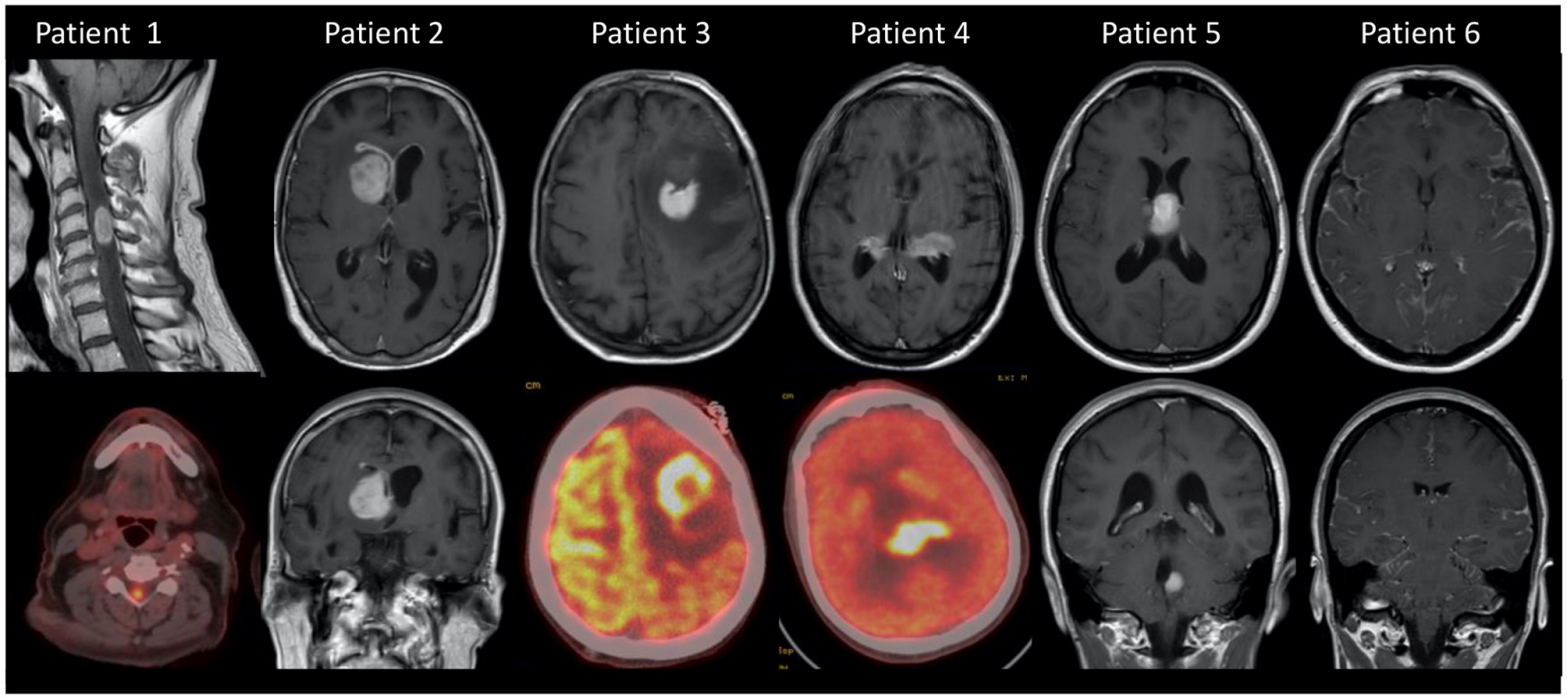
MRI or PET scan images of patients with hematologic CNS disease. Patient 1: SCNSL with spinal cord involvement. Patient 2: SCNSL relapse with leptomeningeal involvement. Patient 3: SCNSL at the frontal left lobe. Patient 4: PCNSL. Patient 5: Multifocal PCNSL. Patient 6: Leptomeningeal diffuse infiltration in a patient with leukemia.
